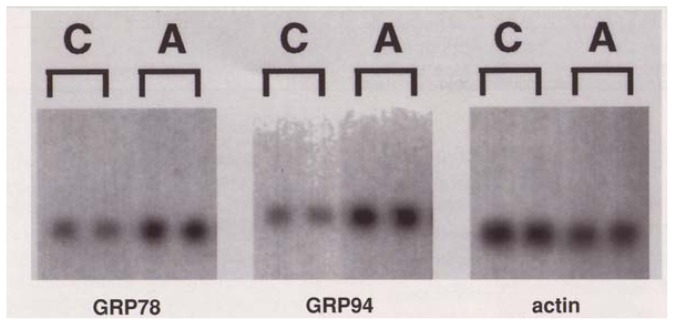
Alcohol increases the expression of two genes encoding the molecular chaperone proteins GRP78 and GRP94 but not of the gene encoding the protein actin. To assess the effect of alcohol on gene expression, mRNA’s isolated from alcohol-treated cells (A) and untreated control cells (C) were incubated with radioactive probes that bind to the specific mRNA’s and which can be detected on x-ray film. The black bands give a visual estimate of the relative amount of the particular mRNA. Alcohol treatment increased the amount of GRP78 and GRP94 mRNA but had no significant effect on the amount of actin mRNA in the cells.
